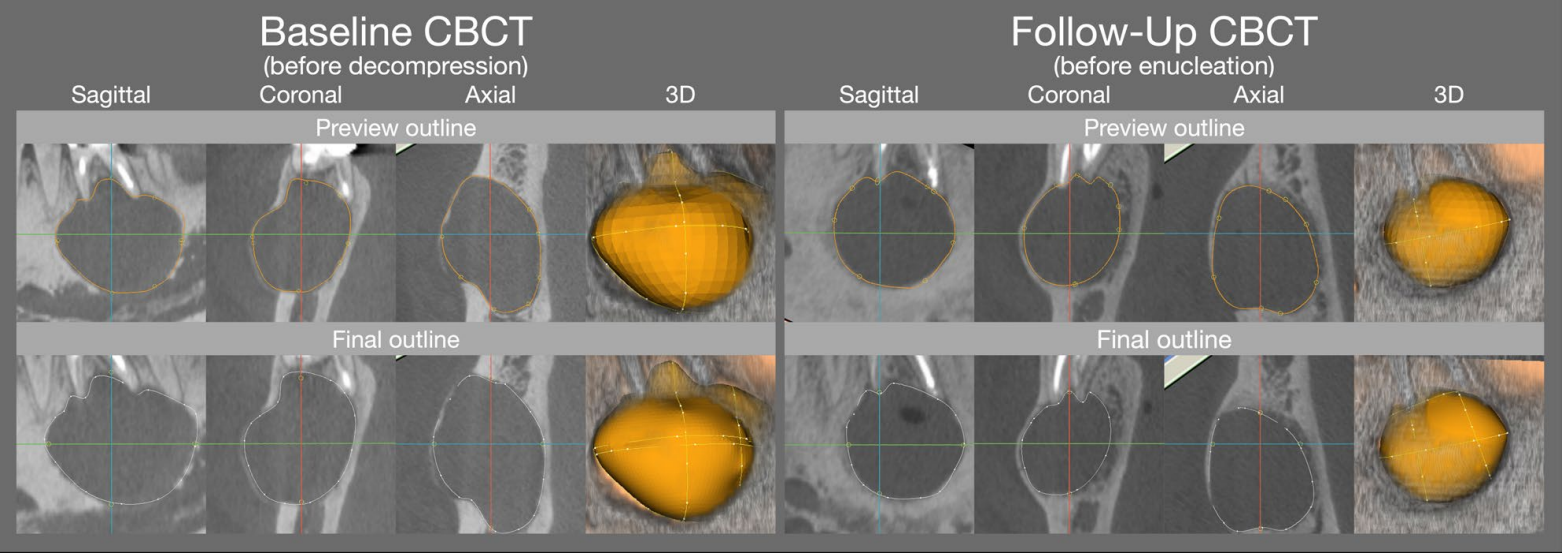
Representative case demonstrating the preview and final outlining on the baseline and follow-up CBCT, facilitating the calculation of the volumetric, surface area and maximum diameter dimensions
